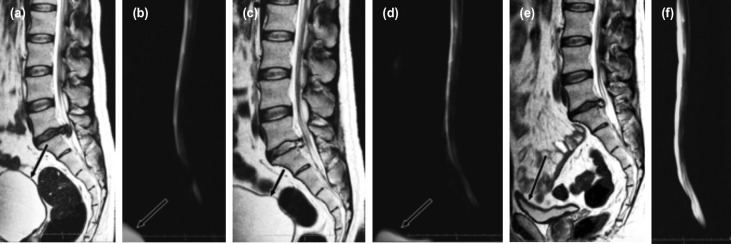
(a, b) MRI imaging in a patient with central big disc, complete myeloblock and CESR (Cauda Equina Syndrome Retention). Arrow showing distended bladder in MRI and myelogram. (c, d) Immediate post-operatively, patient had slow urine stream and needed straining to empty. The MRI showing a complete decompression and a clear myelogram. Arrow showing distended bladder post-operatively in MRI and myelogram as well. (e,f) MRI and myelogram on follow-up ten days shows complete empty bladder (arrow) without any clinical bladder dysfunction.
